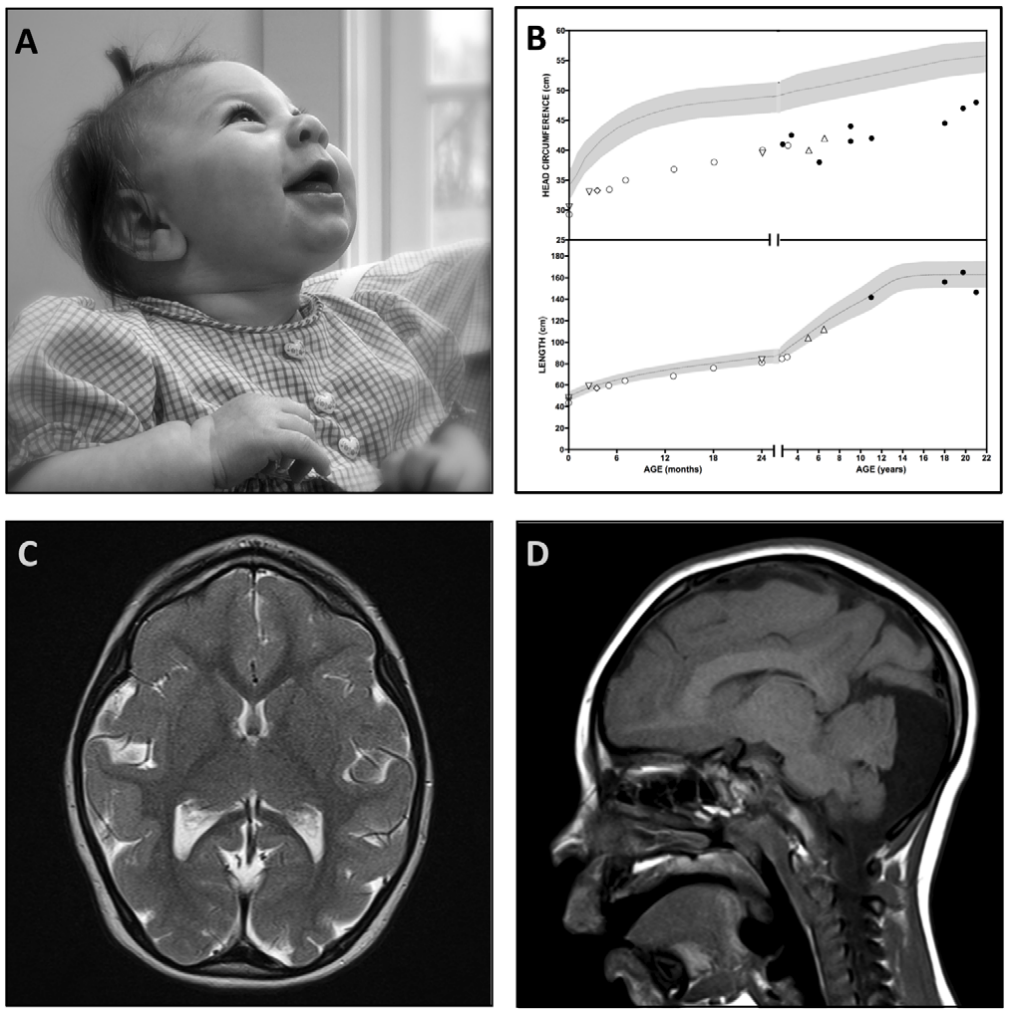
Microcephaly and chorioretinopathy due to a homozygous *TUBGCP6* mutation. (A) An affected infant has marked microcephaly (>4SD below normal), a receding forehead, diminutive anterior fontanelle, and sutural ridging. She has cognitive delay and visual impairment but is socially engaged. (B) Head circumference and length plots for Mennonite microcephaly patients. (C) Brain magnetic resonance imaging (MRI) shows diffuse pachygyria, normal myelination, and (D) a hypoplastic cerebellar vermis.
